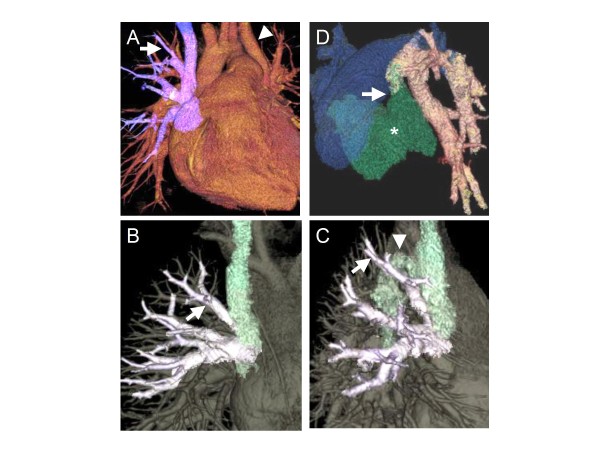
Volume-rendered image multidetector-row computed tomography (MDCT).    A - Blue-colored vessels return to the superior vena cava (SVC). The arrow indicates a high connected pulmonary vein. The arrowhead indicates the left persistent SVC.  B, C - Target vessel-enhanced image derived from MDCT. The arrow indicates the highest SVC site connection, which without this image is at risk of being mistaken for the azygos vein. The arrowhead indicates the azygos vein. D - A target component-enhanced image derived from MDCT. The arrow indicates the anastomosis site of the left atrium appendage with the vertical vein. The asterisk (green) indicates the left atrium.
